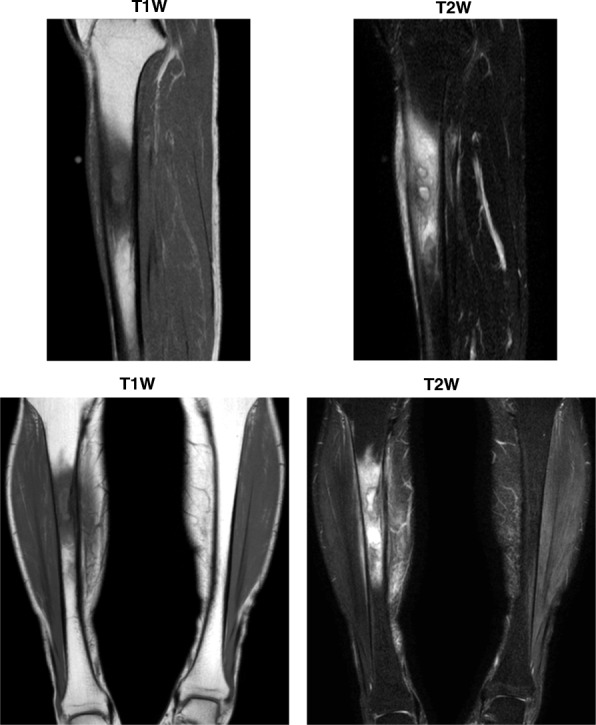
Magnetic resonance imaging showed an abscess and inflammatory change in right lower leg. At the onset of type 2 diabetes mellitus, axial T1-weighted image showed a slightly lower intensity, and axial T2-weighted image showed a markedly higher intensity (*upper panels*). Magnetic resonance imaging showed a spreading of the abscess and inflammatory change in right lower leg after the interruption of therapy at the age of 58 (*lower panels*). *T1W* T1-weighted image, *T2W* T2-weighted image
